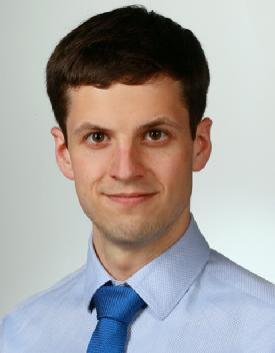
Konrad Pieszko MD, PhD works in the Cardiology Clinic of the University of Zielona Góra, Poland, and currently participates in advanced cardiac imaging fellowship at Sant Pau Research Institute–Hospital de la Santa Creu i Sant Pau, Barcelona. His research integrates artificial intelligence with multimodal cardiac imaging including CT, PET/SPECT, CMR and echocardiography to improve risk stratification.
